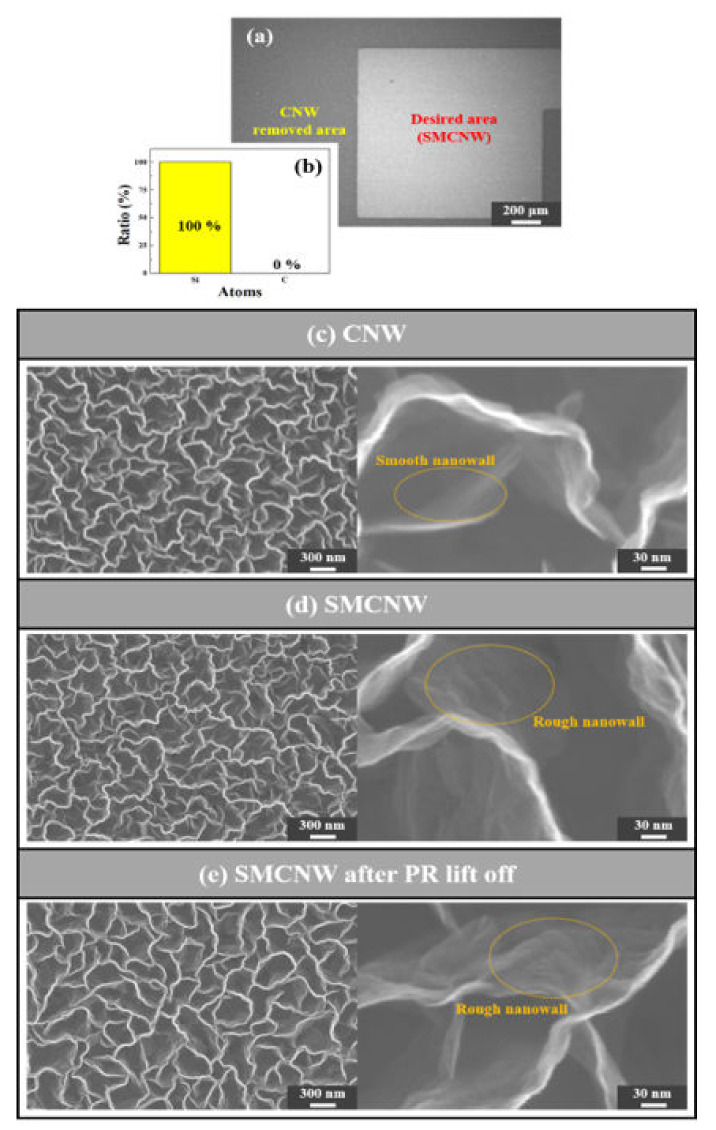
(a) FESEM surface image after applying the top-down surface micromachining technology to the SMCNW. (b) EDS analysis of the area where the CNW was removed through ultrasonic cleaning. FESEM surface image of the (c) CNW, (d) SMCNW, and (e) PR-removed SMCNW.
